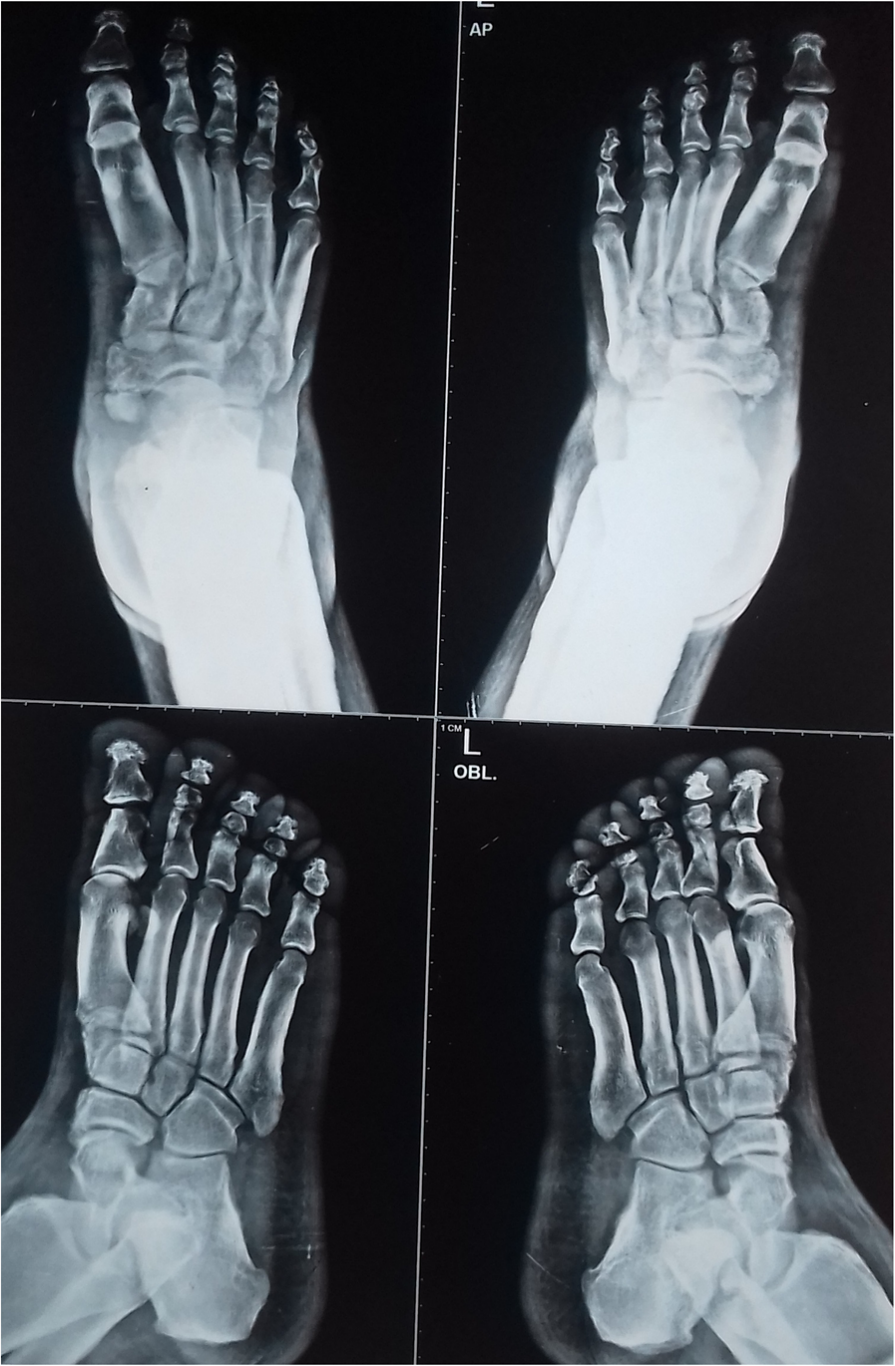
Radiograph of bilateral ankles showing irregular subperiosteal bone formation and cortical thickening of distal tibia, fibula, calcaneum, and talus
